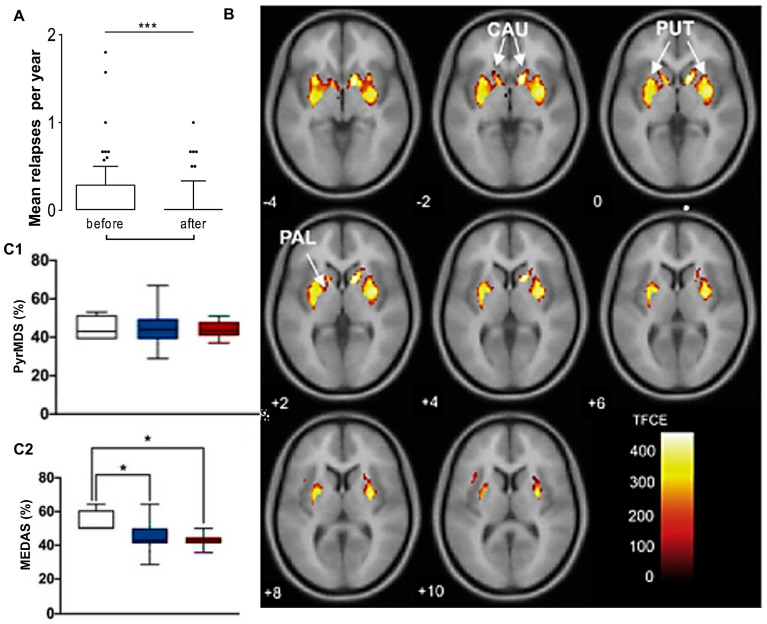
Impact of diet on patients with multiple sclerosis. (A) Long-term supplementation with propionic acid (PA) in 97 patients with MS, where patients were stratified with ARR (annual relapse rate) [304]. Further, it was concluded that 41.2% patients showed improvement while 47.4% remained stable, and 11.3% exhibited increased ARR after PA supplementation (* *p* < 0.001). (B) MRI scans of twenty-two patients supplemented with PA. The results concluded a significant reduction in global and regional volumes (* *p* < 0.05) (C1,C2). Evaluated adherence to the Mediterranean diet in patients with multiple sclerosis [305]. This was assessed using MSSS (MS severity score), MEDAS (Mediterranean Diet Adherence Screener), and PyrMDS (Pyramid-based Mediterranean diet score). Further, pwMS (people with multiple sclerosis) was divided by MSSS: Mild (0–1.7), Intermediate (1.7–5), and Aggressive (>5).
